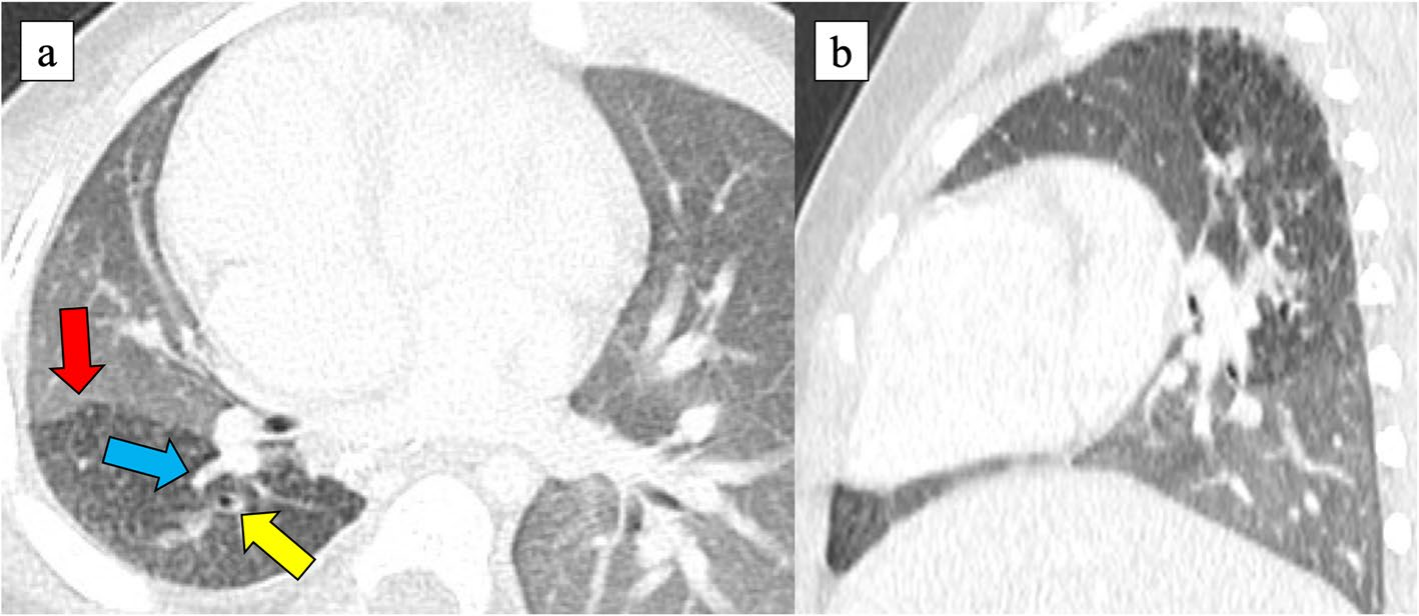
CT scan 3 months postoperatively. It revealed slight overinflation of the preserved affected lung (the S6 lesion) with pulmonary vessels (V6: blue arrow) and bronchus (B6: yellow arrow). The margin of the affected lung could be seen (red arrow). a Axial and b sagittal section
